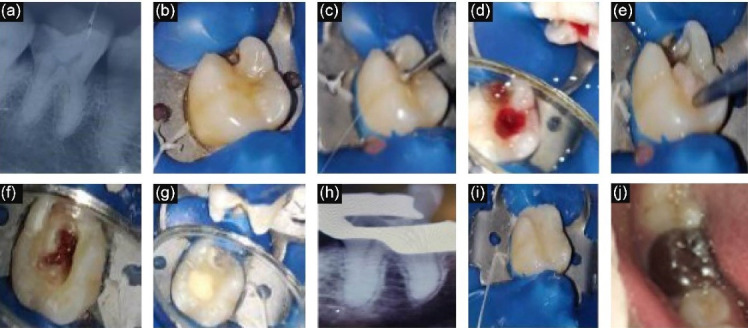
All the included teeth were observed clinically at 1, 3, 6, 9, 12, and 24 months and radiographically at 6, 12, and 24 months. At each follow-up visit, each treated tooth was examined for the following adverse events: pain, swelling, sinus tract formation, tenderness on percussion or palpation, and radiographic evidence of peri-radicular or furcal pathology or root resorption. In cases of either clinical or radiographic failure, Biodentine pulpotomy was designated as a failure, and RCT was undertaken. The cases considered clinical or radiographic failure underwent RCT and were excluded from the study. Such teeth were considered a failure in clinical or radiographic and vice versa. The data obtained were subjected to statistical analyses using the chi-squared test.
